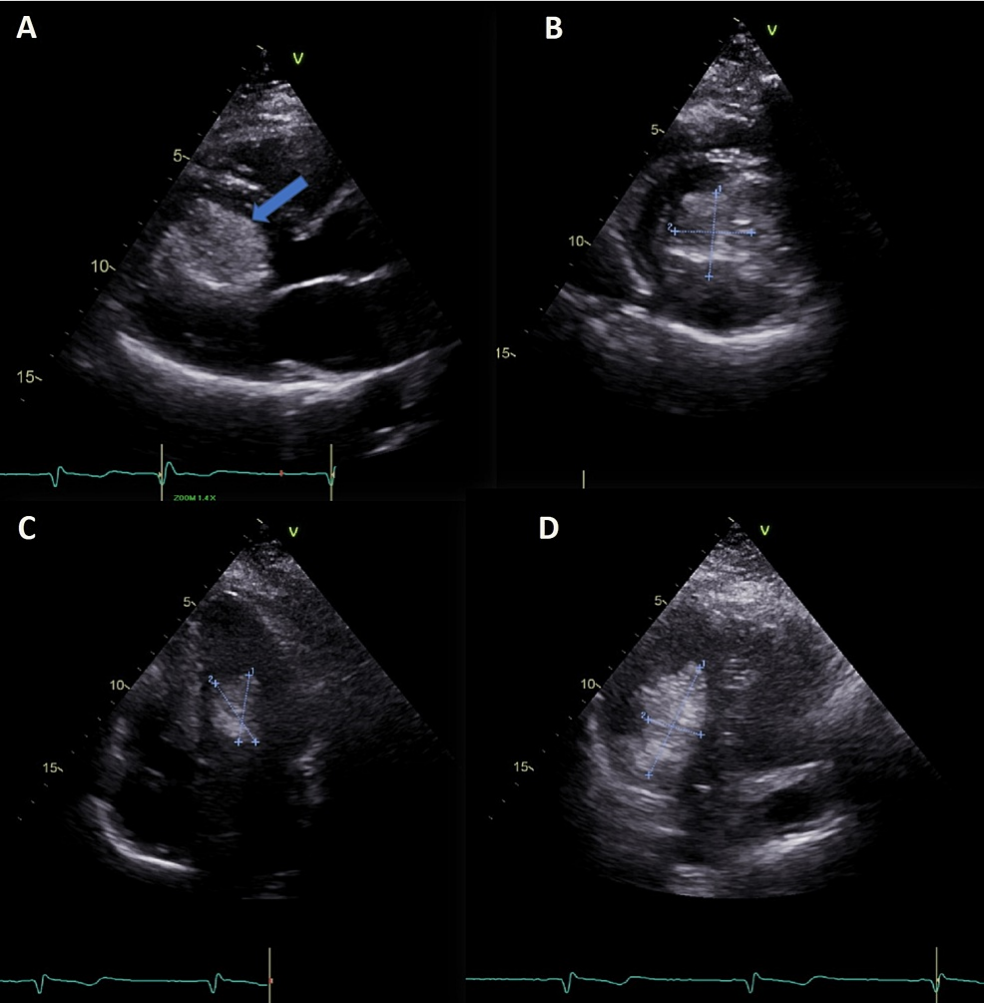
Transthoracic echocardiogram Parasternal long-axis (A), parasternal short-axis (B), four-chamber (C), and three-chamber (D) views showing left-ventricular lipoma (blue arrow).
